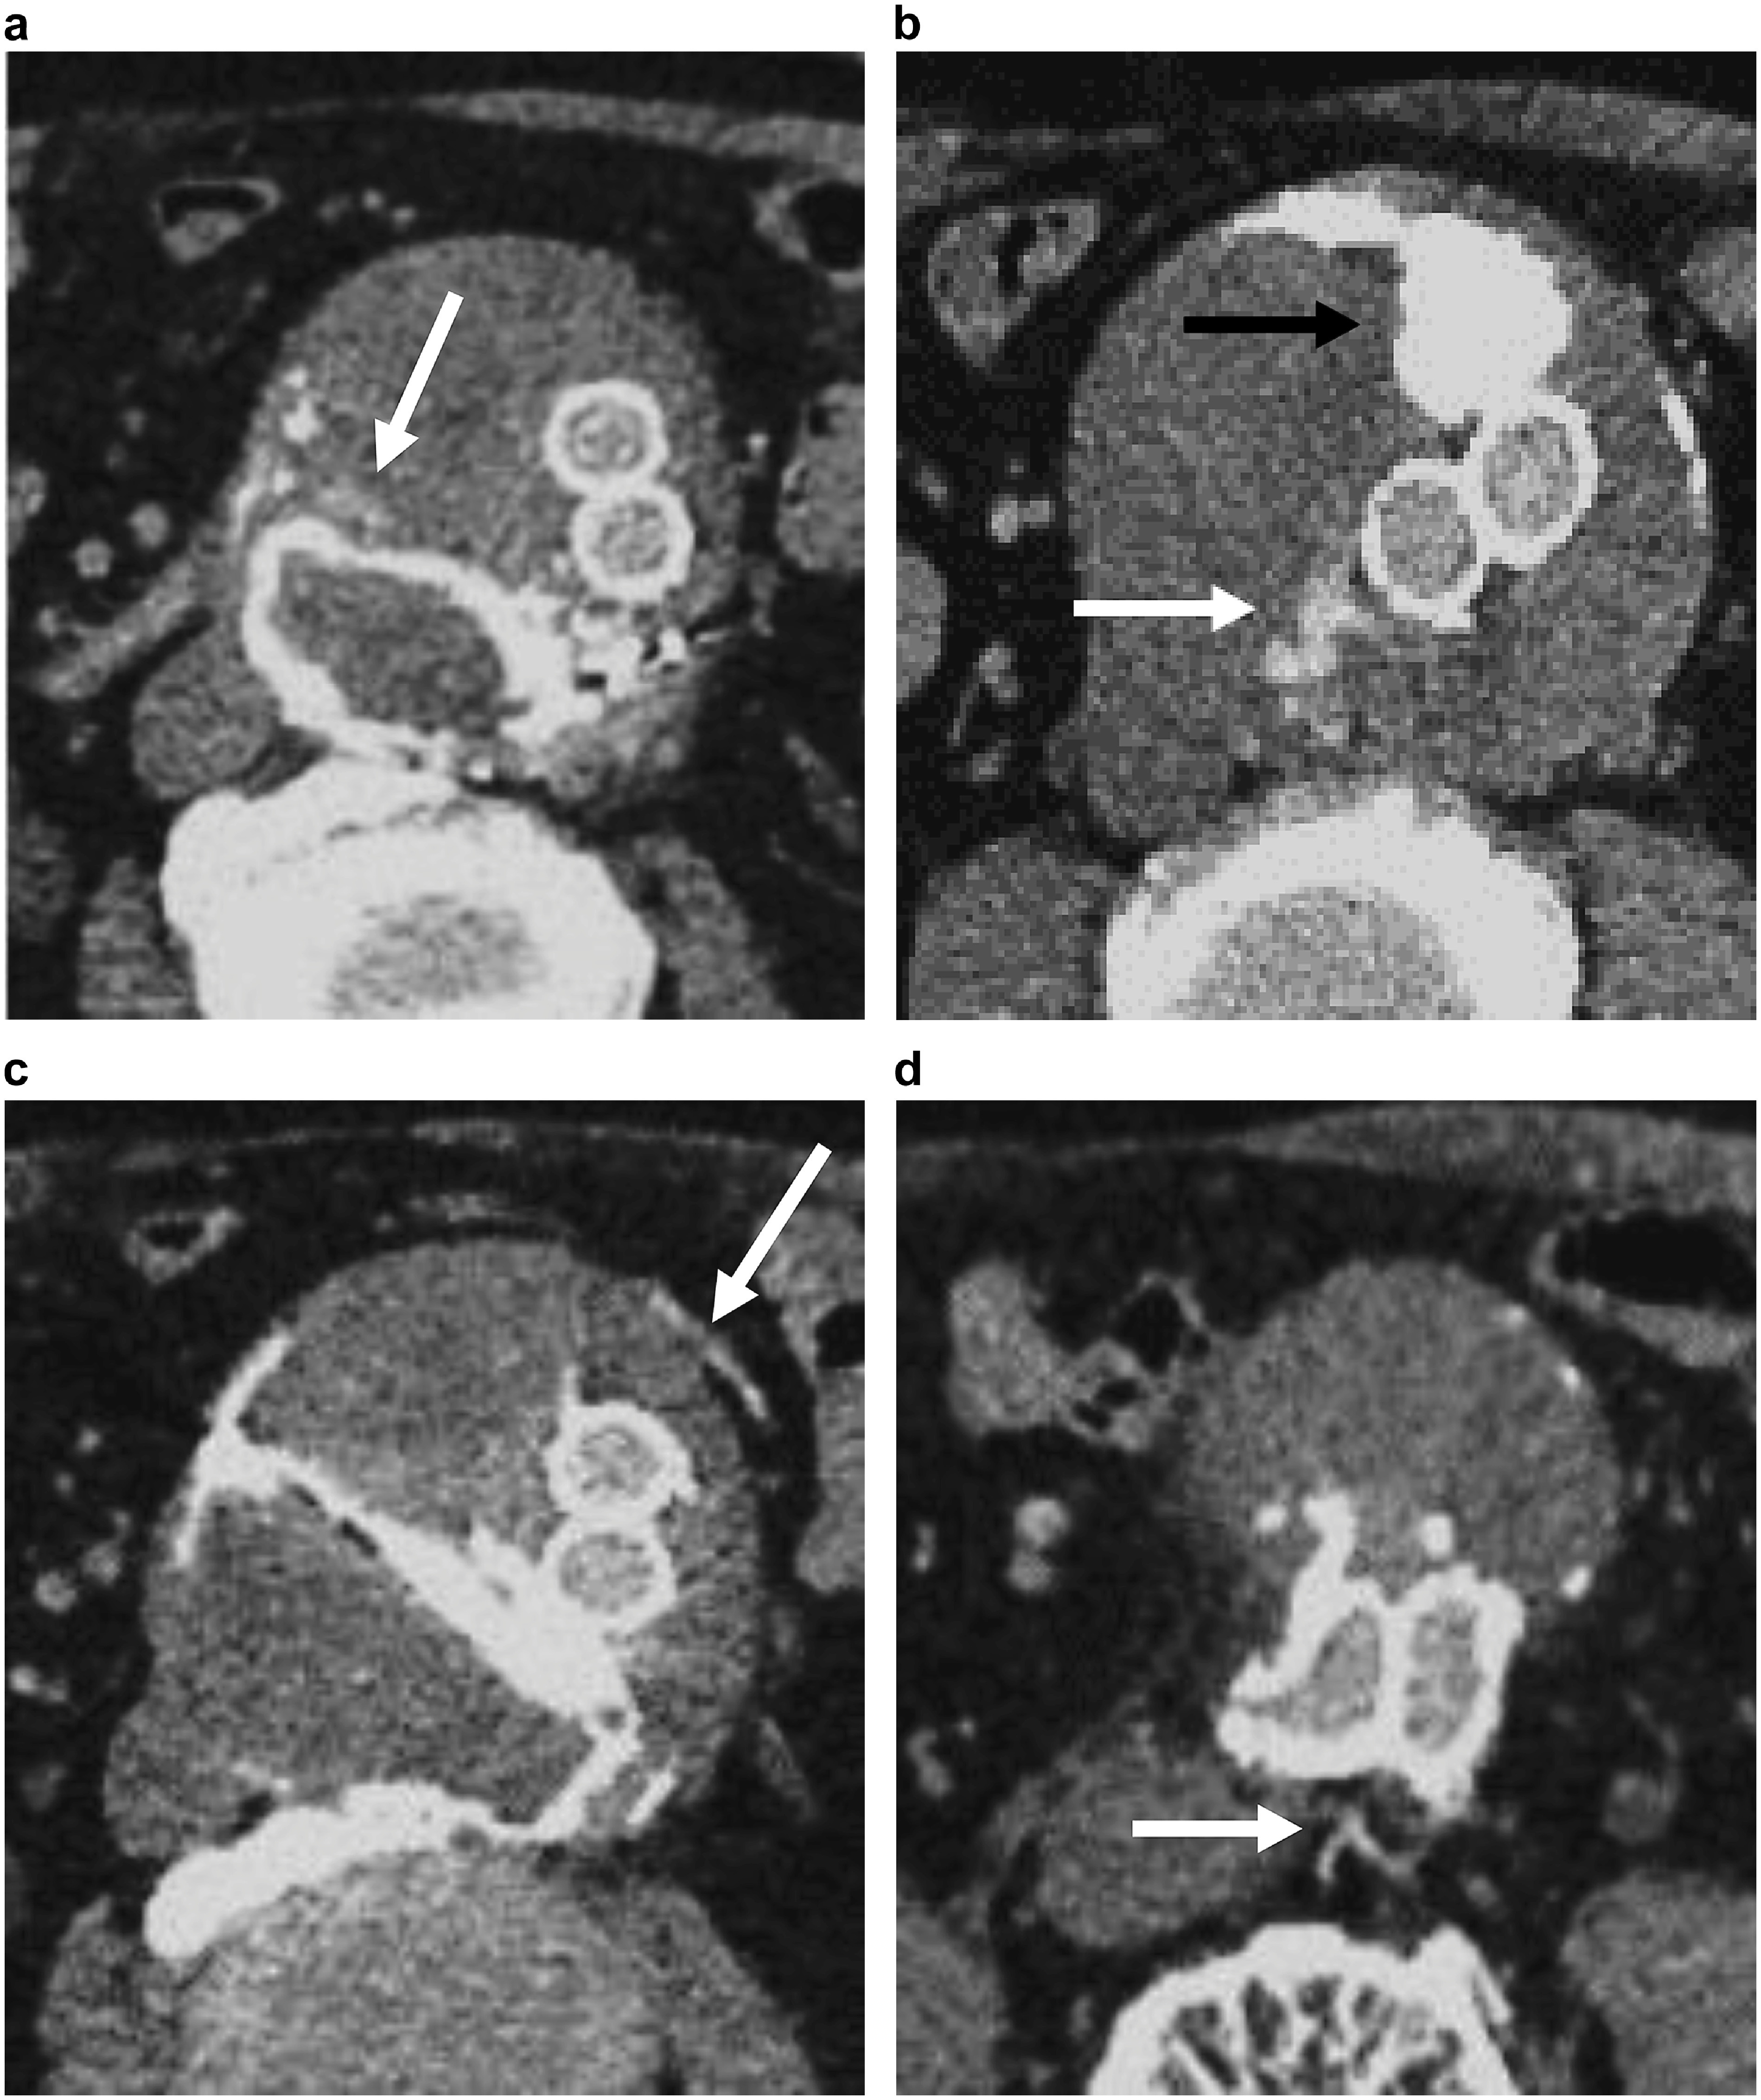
Fig. 1 Contrast-enhanced computed tomography findings 2 years after an endovascular aortic repair. Two endoleaks (white arrow) were observed: (a) the one with contrast medium leakage on the right side was not connected to any vessel and was not classified into a particular type and (b) the other one on the posterior side of the aneurysm originated from the third lumbar artery and was classified as type II. (c) The inferior mesenteric artery and (d) the median sacral artery were patent (white arrow). The black arrow indicates the n-butyl-2-cyanoacrylate in the sac.
